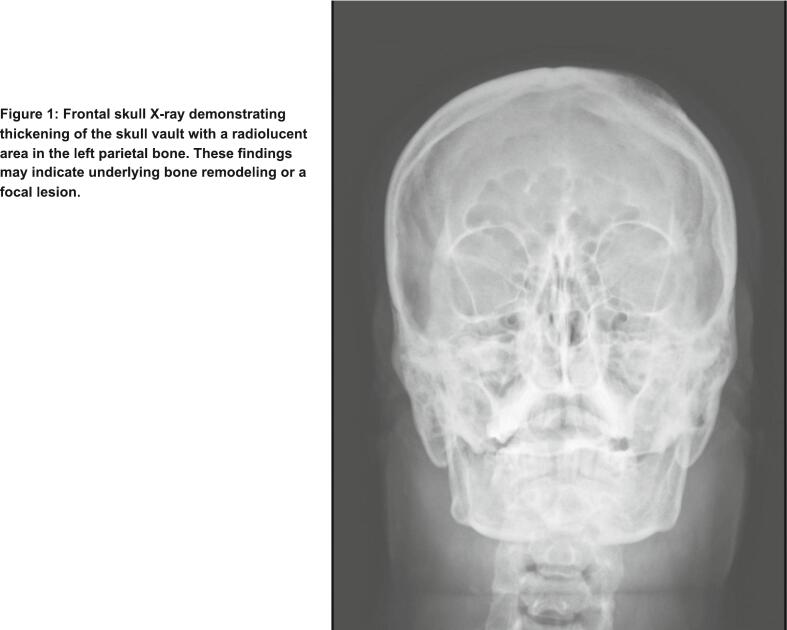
Frontal skull X-ray demonstrating thickening of the skull vault with a radiolucent area in the left parietal bone. These findings may indicate underlying bone remodeling or a focal lesion.
